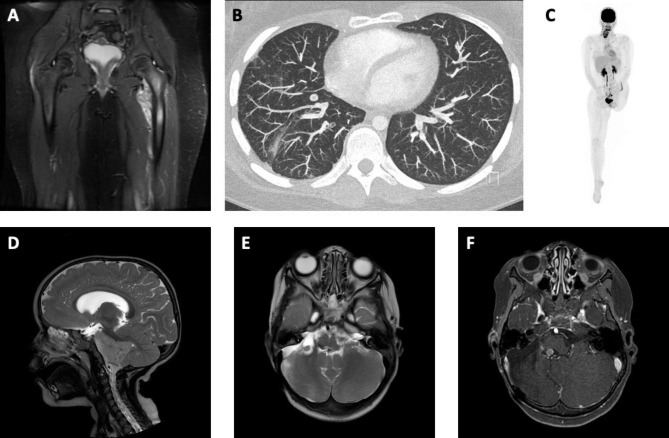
Magnetic resonance imaging (MRI), computed tomography (CT) and positron-emission tomography (PET). CR1: A Coronal T2-weighted MRI of the femur and pelvic region demonstrating the tumor of the left proximal femur. B CT scan demonstrating a single pulmonary metastasis. C PET scan demonstrating a large metastasis at the back and multiple cervical und nuchal metastases. CR2: D Sagittal T2-weighted MRI demonstrating the tumor of the posterior fossa encircling the brain stem. E Axial T2-weighted MRI demonstrating first local relapse. F Axial T2-weighted-Fluid-Attenuated MRI demonstrating second local relapse
